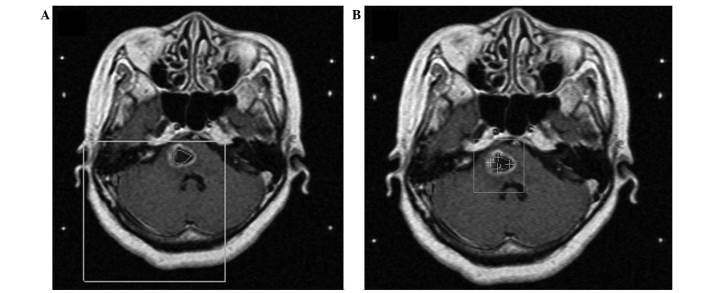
Magnetic resonance images obtained one week following stereotactic aspiration surgery. The tumor decreased in size to 18×15×14 mm (volume, 1.9 cm^3^). (A) Axial T1-weighted images. (B) Axial T1-weighted images showing dose planning of the tumor for gamma knife radiosurgery.
